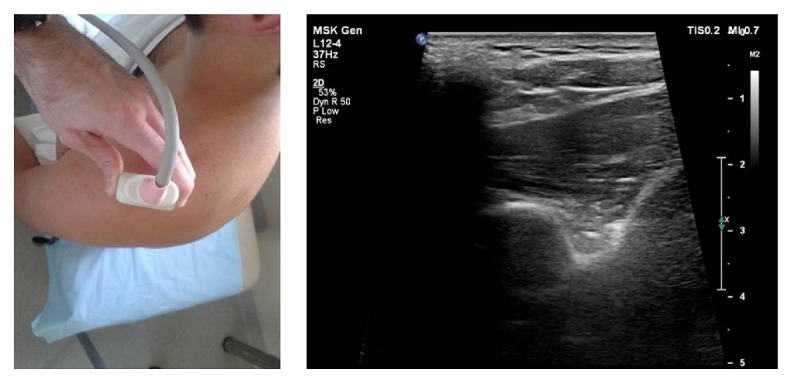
Ultrasonographic investigation of the suprascapular notch region (stage III of protocol).
